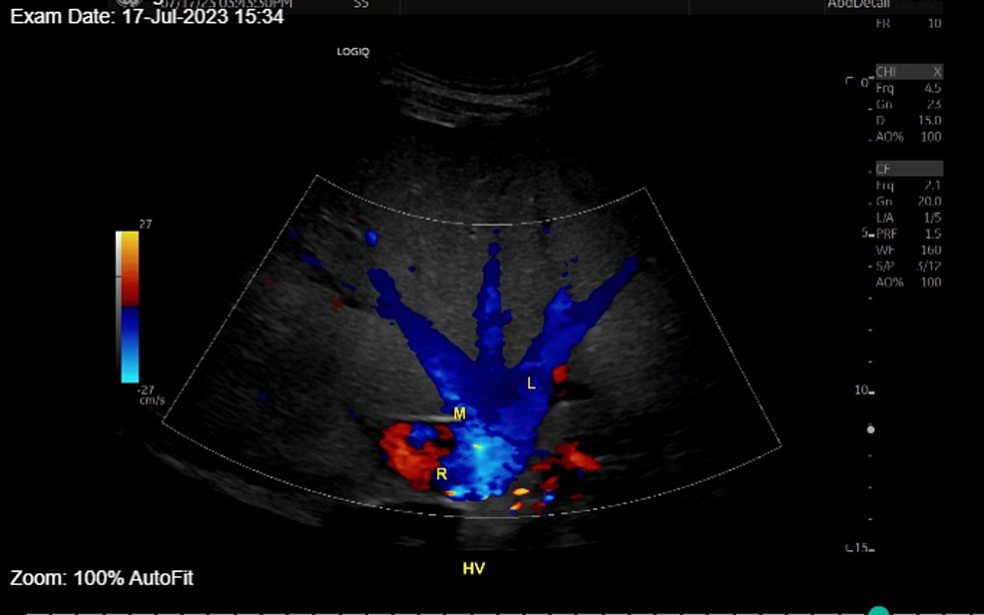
Abdominal ultrasound of the liver demonstrating blood flow in the branches of HV and IVC. Multiple grayscale and color Doppler images of the IVC, common iliac veins, and hepatic veins.
